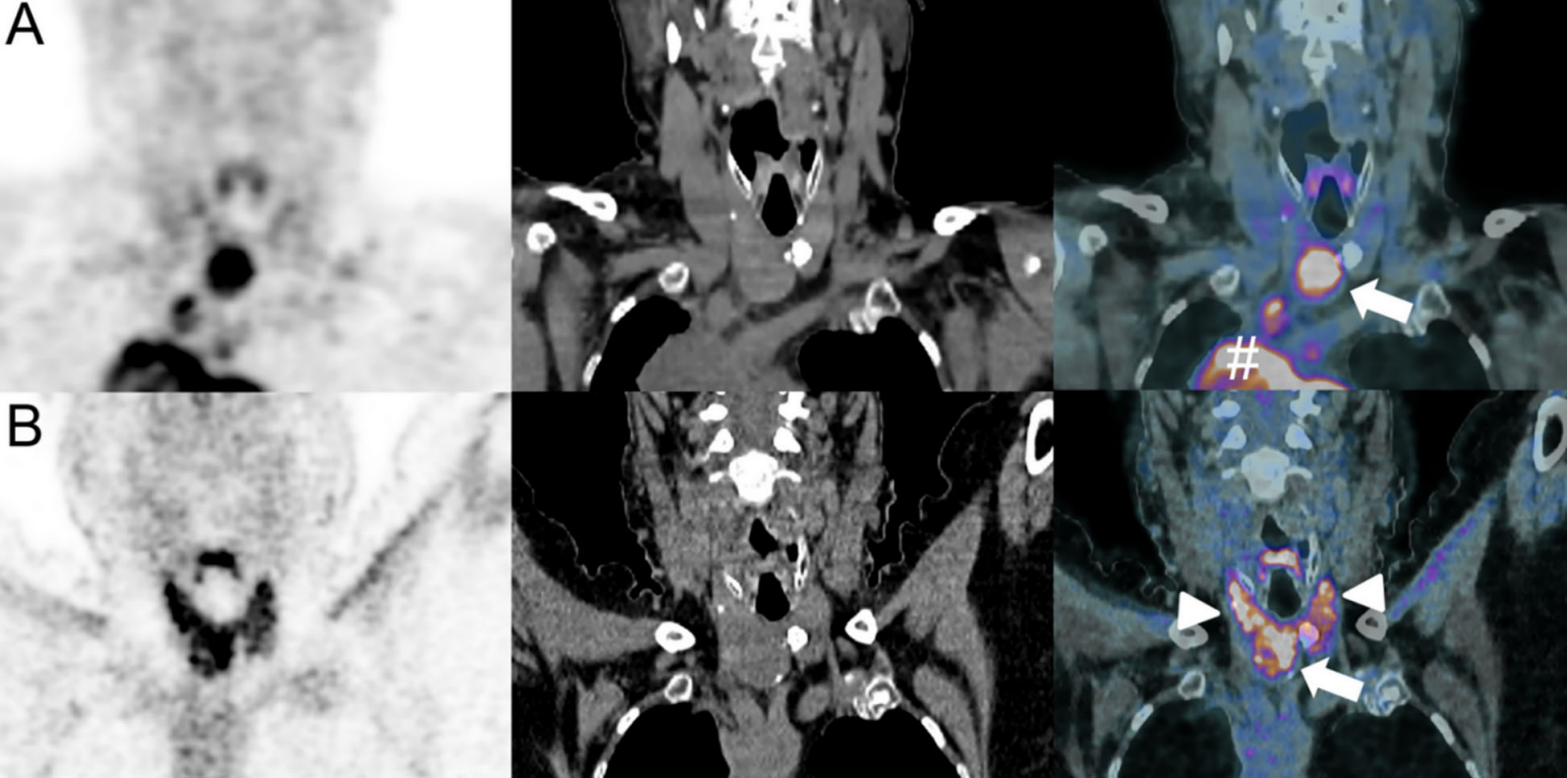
Immune-related thyroiditis in a 50-year-old female with lower leg Meckel-cell carcinoma who developed mediastinal metastatic spread as shown on baseline ^18^F-FDG-PET/CT [coronal PET, CT and merged PET/CT images, # (A)]. A thyroid nodule was also present [arrow, fused PET/CT image (A)]. Pembrolizumab (anti-PD-1) was administered. Follow-up 18F-FDG-PET/CT at 6 months showed disappearance of mediastinal disease and decrease in metabolic activity of the thyroid nodule [arrow, fused PET/CT image (B)]. However, a marked increase in thyroid activity was also evident, consistent with a thyroiditis [arrowheads, fused PET/CT image (B)].
